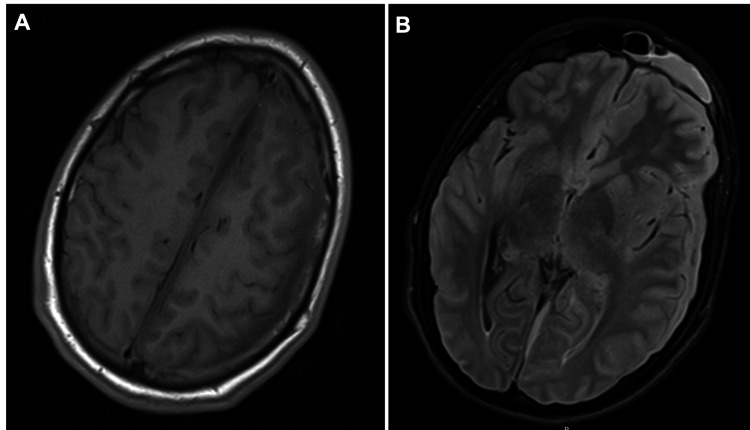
Non-contrast-enhanced cranial MRI at admission. (A) Axial T1 with a subtle extra-axial left parafalcine subdural empyema. (B) Axial FLAIR with an air-fluid level on the left frontal sinus consistent with acute sinusitis. Notice the left frontal subdural empyema causing a slight anterior midline shift. FLAIR: fluid-attenuated inversion recovery; MRI: magnetic resonance imaging
